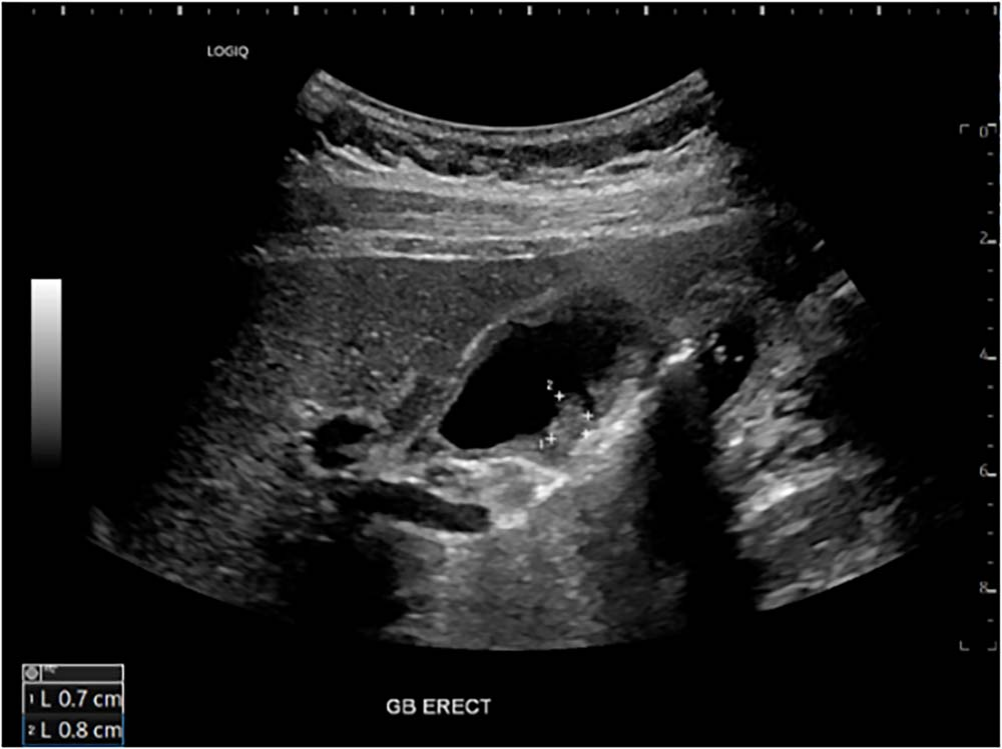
Preoperative ultrasound showing gallbladder polyp and wall thickening.
